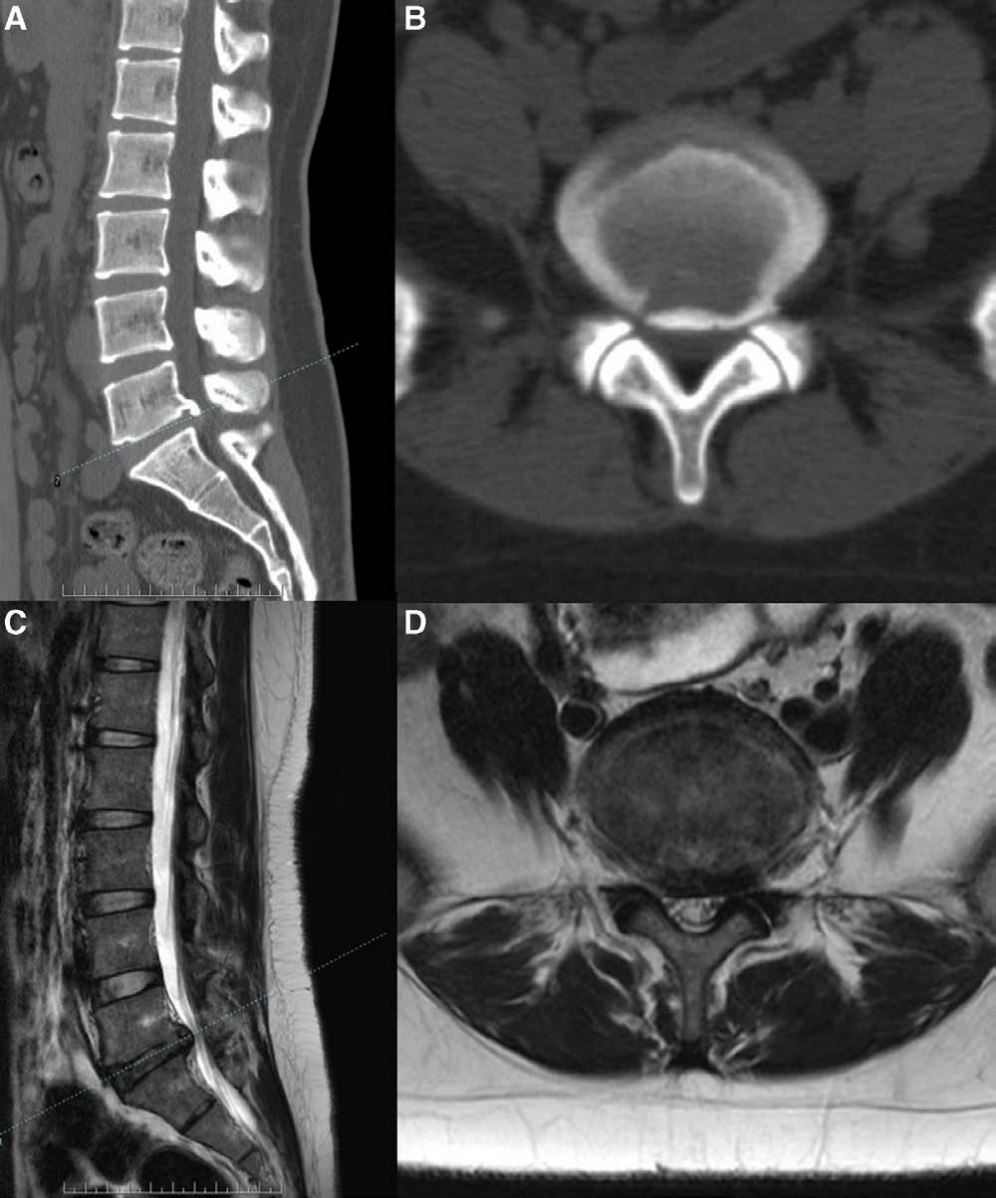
A 21-year-old female presented with low back pain and a 3-month history of painful numbness radiating to the right lower limb. (a, b) Preoperative CT showed calcified disc herniation at the L5-S1 level. (c, d) Preoperative MRI showed disc herniation dominant to the right side. CT = computed tomography, MRI = magnetic resonance imaging.
